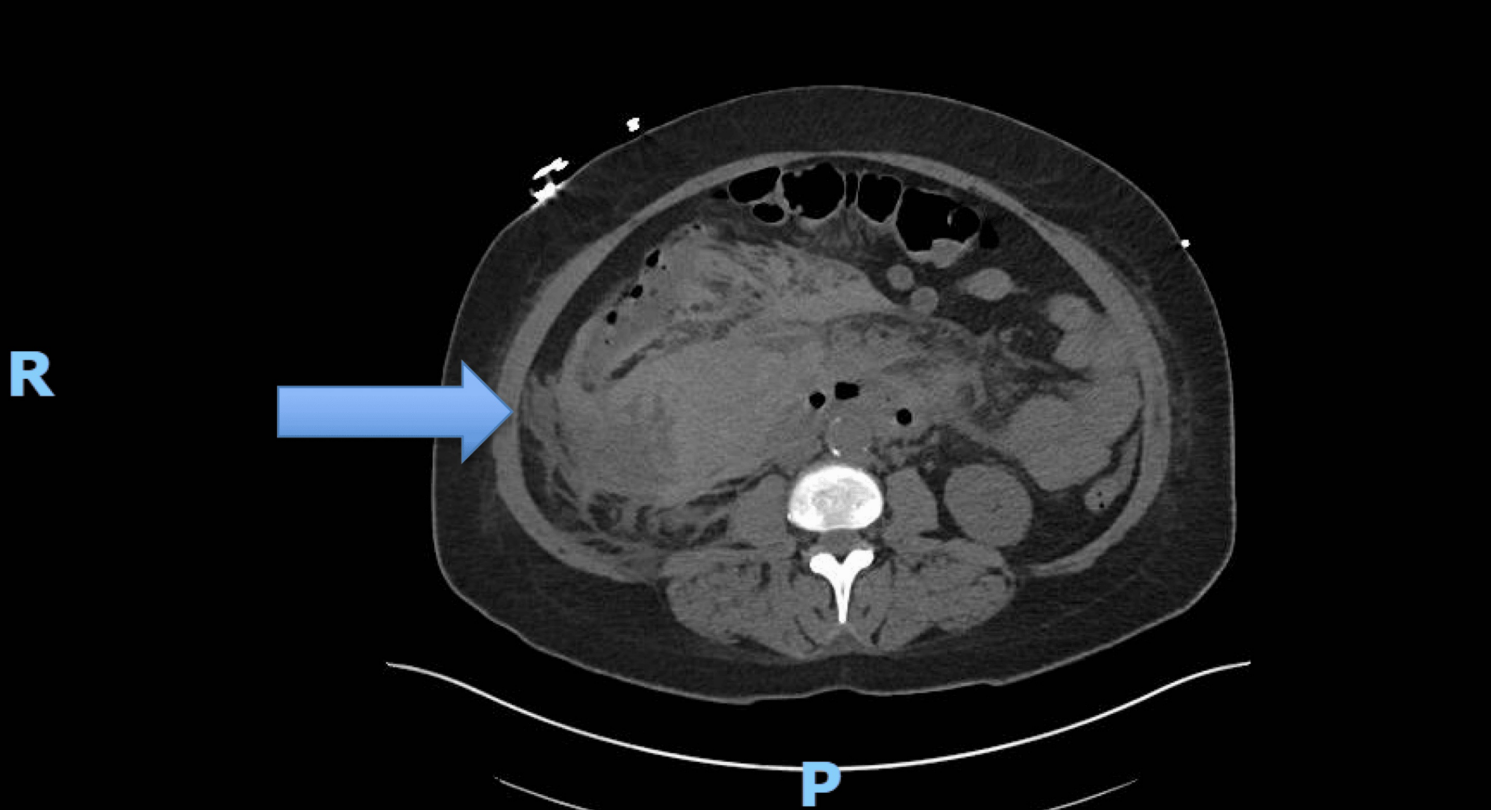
Axial CT image of the abdomen showing a large right retroperitoneal hematoma. The blue arrow indicates hematoma.
